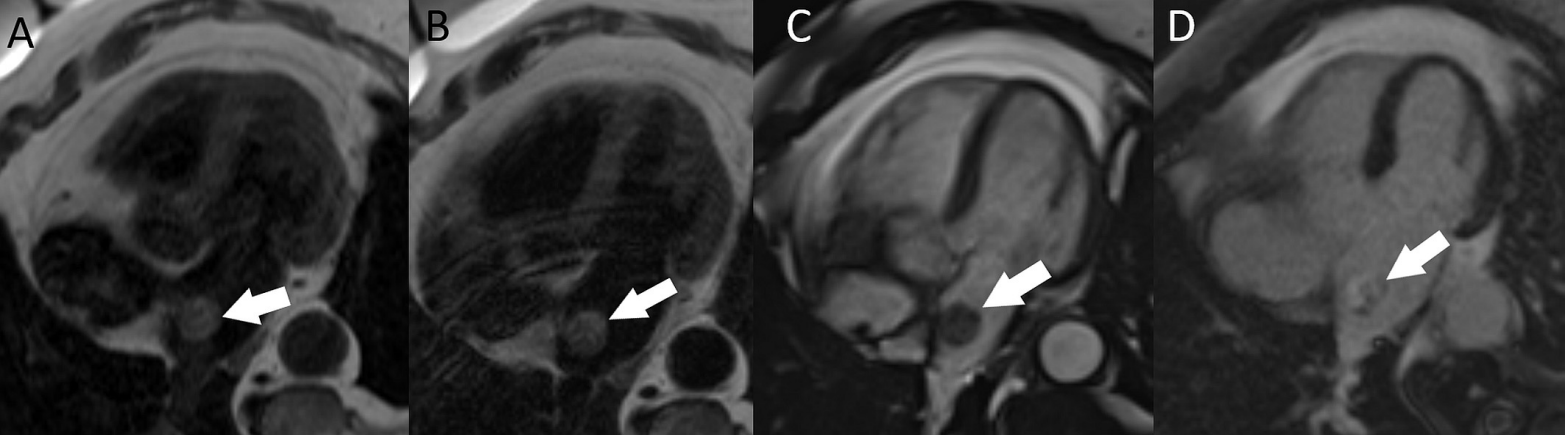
Cardiac MRI in a patient with left atrial myxoma
